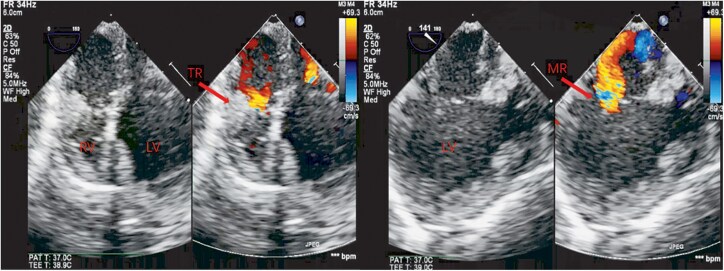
Intraoperative transoesophageal echocardiography. Note: LV = left ventricle; RV = right ventricle; TR = tricuspid regurgitation; MR = mitral regurgitation.
